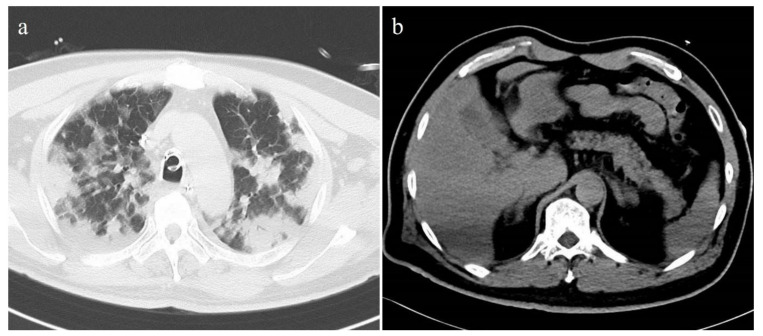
Unenhanced axial CT images of a 51-year-old male patient with severe COVID-19 pneumonia. (a) Chest CT showed extensive consolidations in both lungs. The lung severity score was 3.8. (b) Pancreatic steatosis and fatty liver were seen on the abdominal CT. The CT-FVF of the pancreas was 28.4% and the CT attenuation value of the liver was 46.9 HU.
